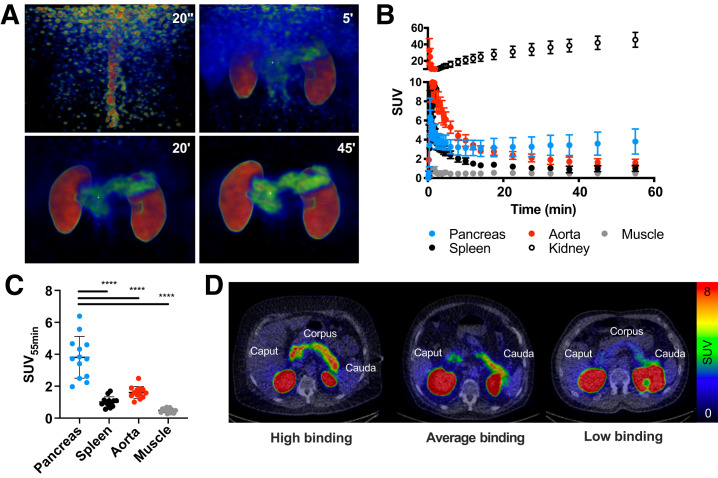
Abdominal biodistribution of ^68^Ga-exendin4 in humans with T2D. (A and B) ^68^Ga-exendin4 was rapidly distributed, followed by washout from most tissues except pancreas and kidneys (average of 13 individuals). Representative maximum-intensity-projection PET images are shown. (C) There was strong variability in pancreas binding, which was not reflected in other tissues. (D) Representative transaxial PET/CT images demonstrate high, average, or low pancreas binding of ^68^Ga-exendin4. indicates *P* < 0.0001.
